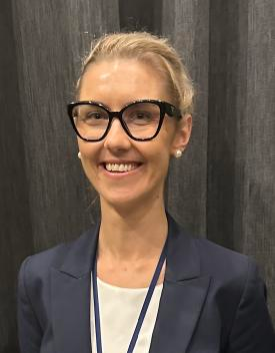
Dr Stephanie Sargent is a cardiologist currently undertaking a fellowship in echocardiography at The Prince Charles Hospital in Queensland, Australia. She completed an undergraduate degree in Biomedical Science at University of Sunshine Coast followed by the completion of Bachelor of Medicine/Surgery at James Cook University, Australia. She completed a Masters in Internal Medicine at the University of Sydney.
